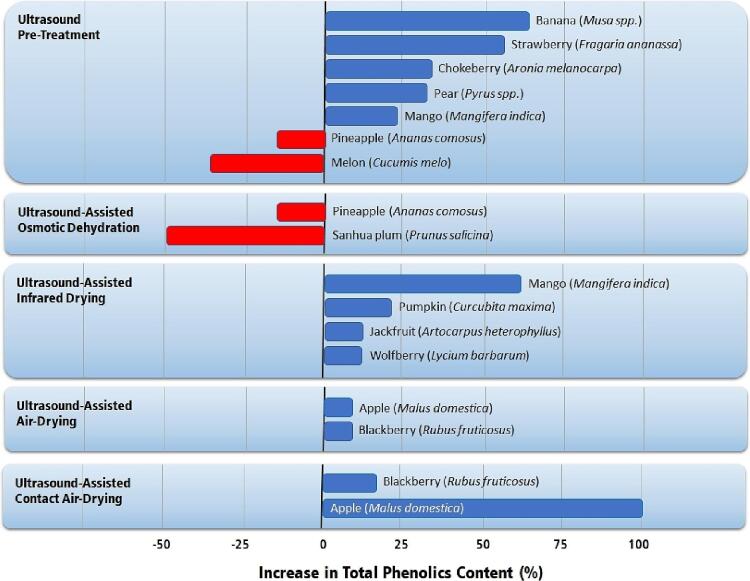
Highest increase in total phenolics content in fruits by applying ultrasound technologies compared to conventional air-drying [24], [38], [39], [92], [95], [97], [98], [99], [100], [102], [103], [104], [117], [118], [119].
